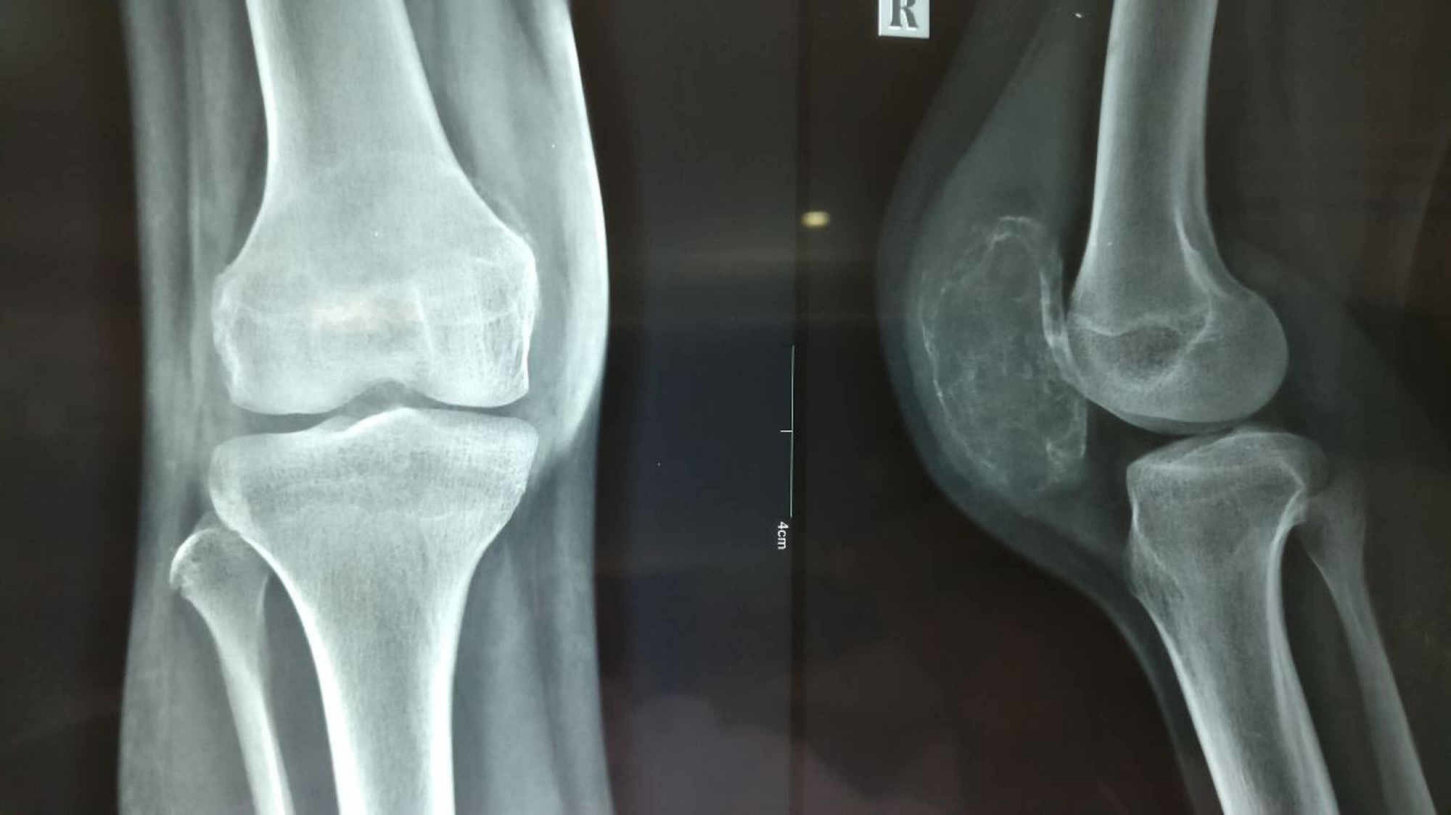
Radiograph of the right knee showing expansile lytic lesion of the whole patella
